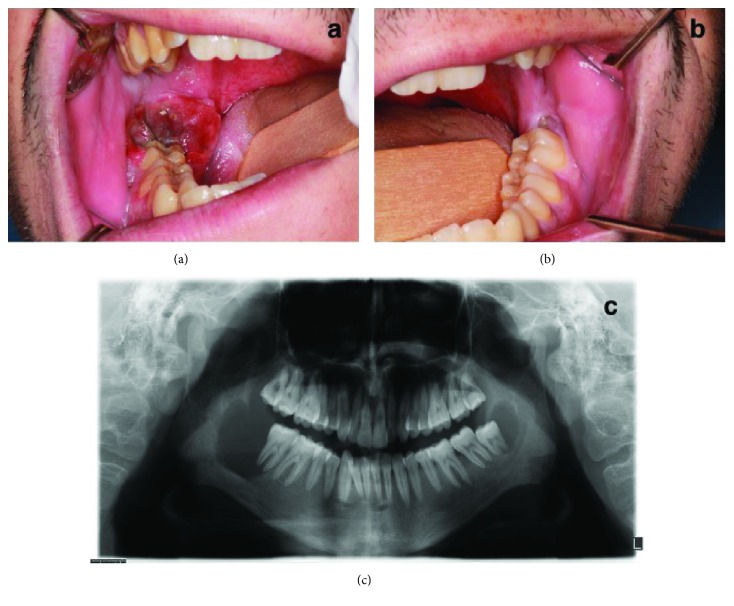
(a) Initial intraoral appearance of the brown tumor (right side). (b) Initial intraoral appearance of the brown tumor (left side). (c) Diagnostic panoramic radiography of bilateral brown tumors.
